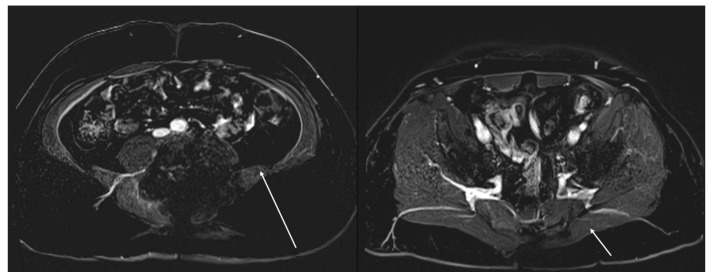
Postoperative MRI indicating asymmetric tissue perfusion. Postoperative MRI scans demonstrating asymmetry in contrast filling (white arrows), suggestive of a possible mismatch in tissue perfusion. The left image reveals reduced perfusion in the postoperative bed, while the right image shows more uniform perfusion. The asymmetry indicated by the arrows may reflect tissue ischemia or a compromised postoperative blood supply.
